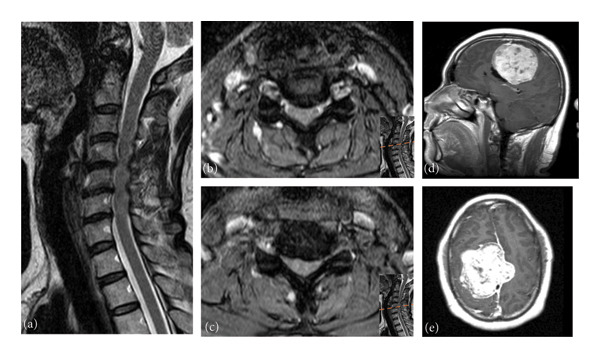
Case 1: Images of cervical magnetic resonance imaging. (a) Sagittal view showing degeneration and ventral spinal cord compression at C4/5, C5/6, and C6/7. (b) Axial views of C4/5. (c) Axial view of C5/6. (d) Sagittal view of brain MRI. (e) Axial view of brain MRI revealed a huge lobulated and well‐defined brain tumor situated in the right frontoparietal parafalcine area, contributing to midline shifting and focal edema.
